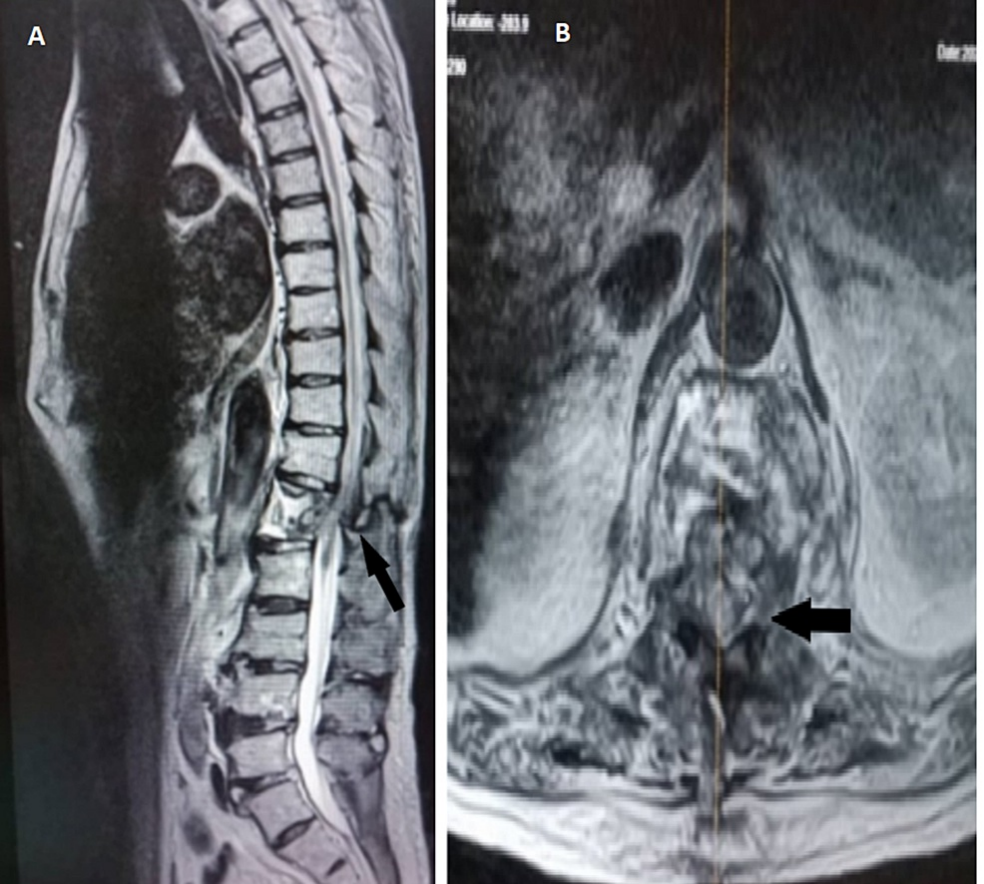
MRI showing intradural hematoma causing spinal canal stenosis and cord edema (black arrow)
